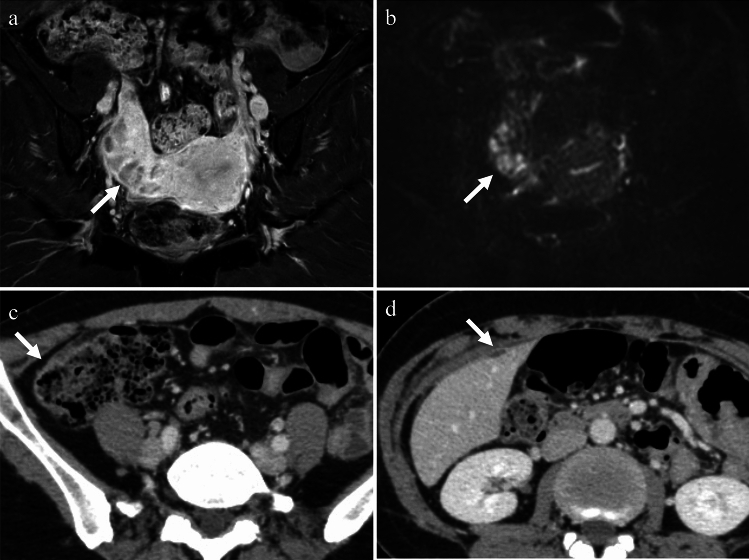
Woman in her 30 s with fever and right lower quadrant pain who tested positive for Chlamydia. a Fat-saturated contrast-enhanced T1-weighted image showing swollen and well-enhanced right adnexa (arrow), along with the presence of multiple cysts. b Cyst contents exhibit markedly restricted diffusion (arrow), suggesting the presence of pus and tubo-ovarian abscesses on diffusion-weighted image. c Thickening and enhancement of the peritoneum (arrow) in the right lower abdomen on contrast-enhanced CT are indicative of peritonitis. d Localized fluid accumulation with a peripheral enhancing effect at the margins of the hepatic surface (arrow) is observed on contrast-enhanced CT, suggesting the presence of an abscess
